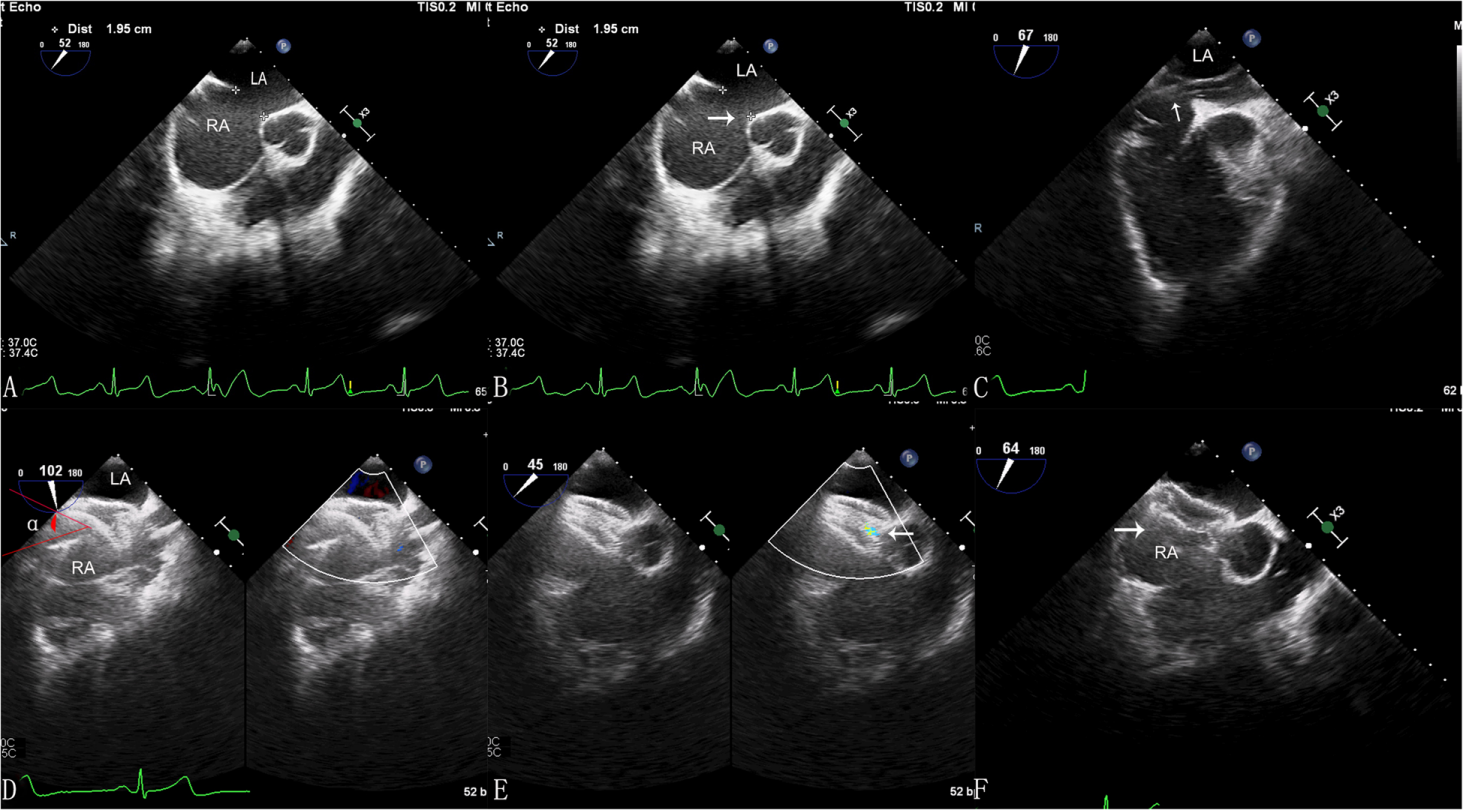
Two-dimensional transoesophageal echocardiography(TEE) imaging (a-f). a Two-dimensional image shows a larger defect. b There is not well rim around the defects(white arrow). c The delivery catheters crossing the defect. d The delivery catheters and atrial septal form an angle(α°). e A mild residual shunt(white arrow) after completely released device. f The occlusion is finished and the device keep perfect form(white arrow).LA:left atrium; RA:right atrium
